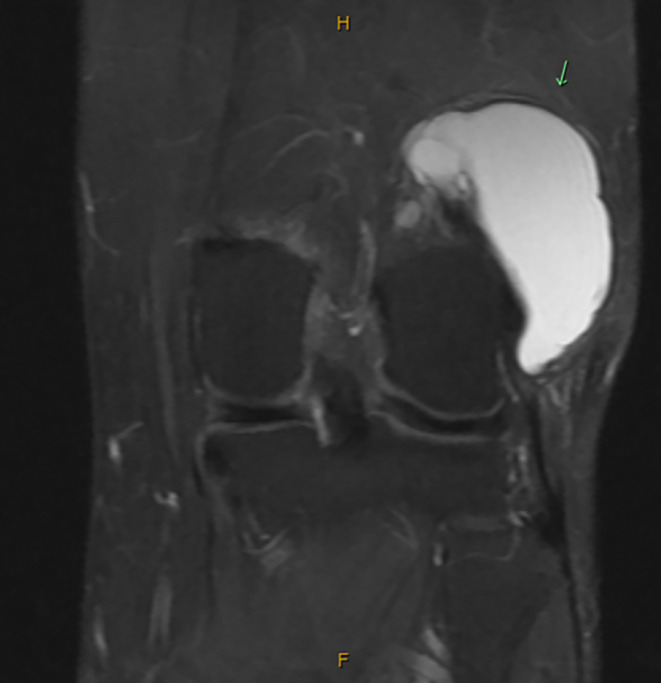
T2 coronal view MRI without contrast of the left knee depicting multiloculated cystic lesion deep to the IT band.
